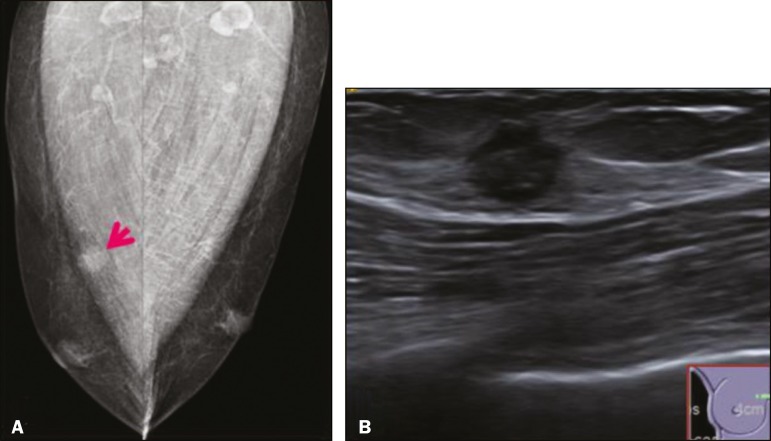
Digital mammography, in mediolateral oblique views (A), and ultrasound (B) in a 57-year-old male (case 1), showing a mass with indistinct margins in the upper inner quadrant of the right breast. The ultrasound shows a heteroechoic mass with microlobulated, fine spiculated margins, adjacent to the pectoralis major muscle. Pathology confirmed the diagnosis of GCT.
